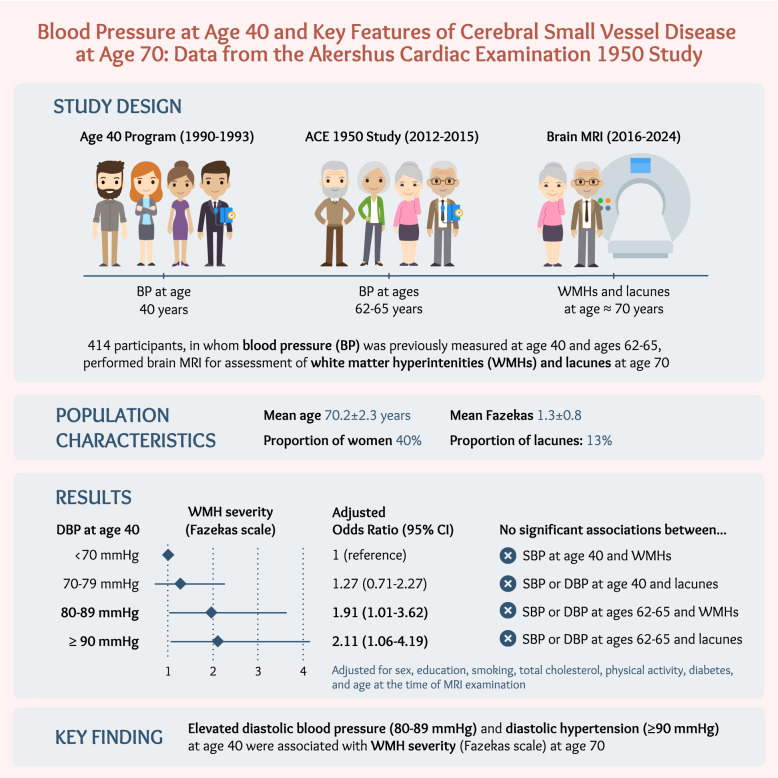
Figure developed by the authors using venngage.com with a license to use, reproduce and distribute worldwide. ACE, Akershus Cardiac Examination. BP, blood pressure. DBP, diastolic blood pressure. MRI, magnetic resonance imaging. WMHs, white matter hyperintensities.
